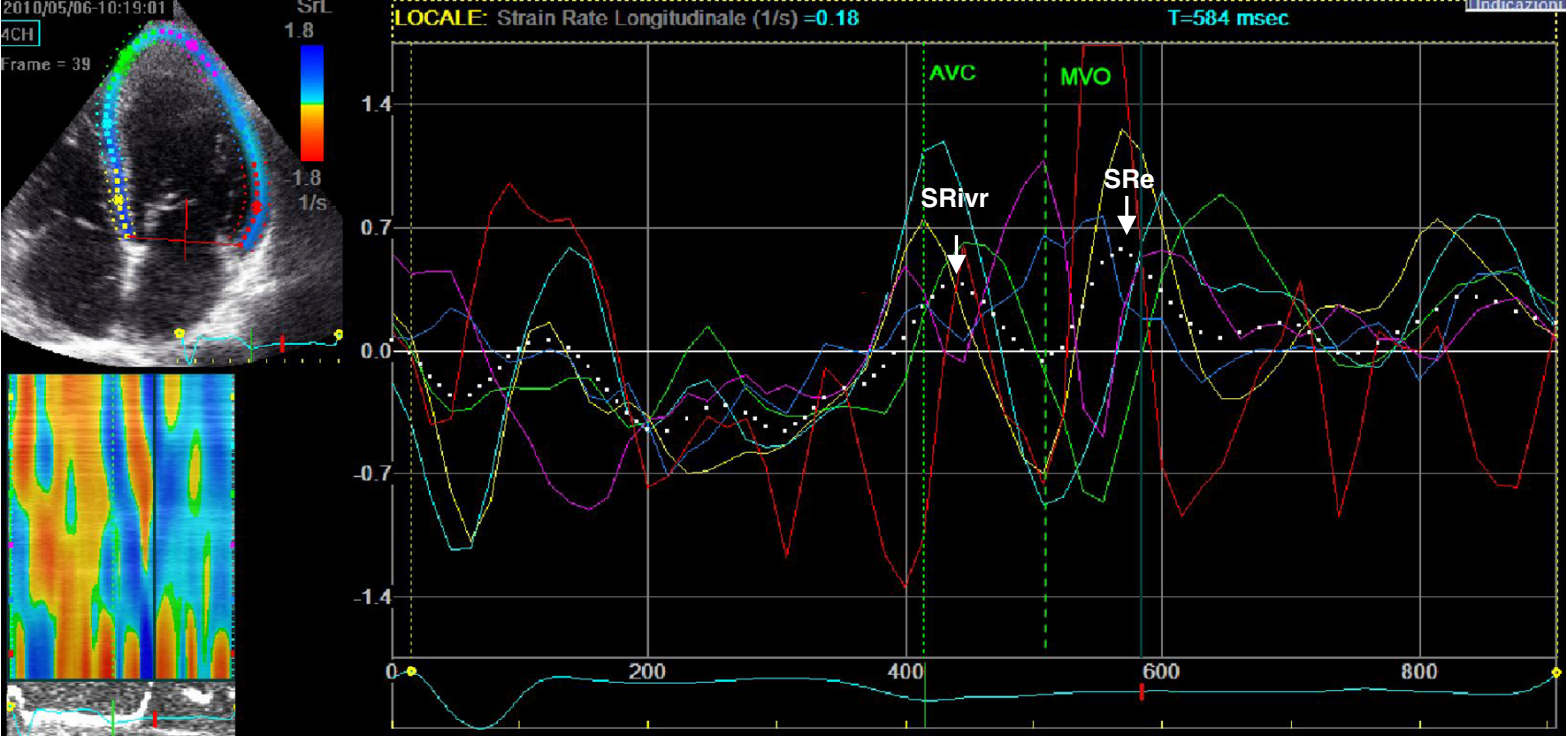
Strain rate analysis using spleckle-tracking echocardiography applied to a 4-chamber view in a patient. The mean derivative curve of strain (*white*, *dotted line*) shows two diastolic peaks. The *first peak* represents the global peak strain rate during isovolumetric relaxation (SR_ivr_), while the *second peak* represents strain rate during early ventricular filling (SR_e_). *AVC* aortic valve closure valve closure, *MVO* mitral valve opening
